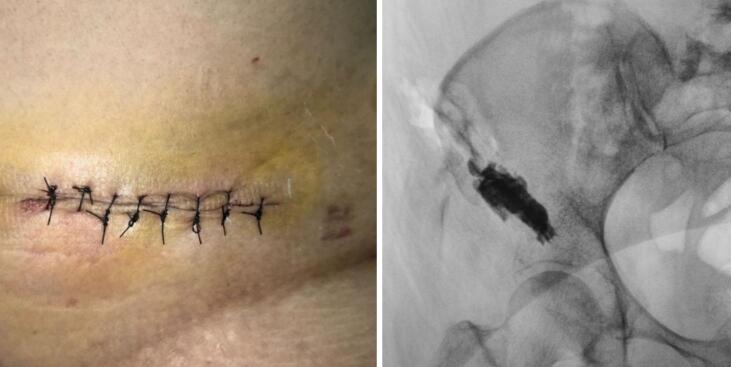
Post operative wound care with 5 cm incision (left). Radiograph post ablation, osteoplasty, and cementoplasty (Right).
